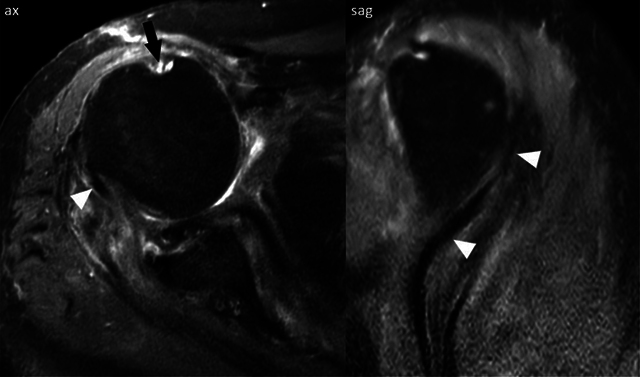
Axial and sagittal fat-saturated proton density-weighted MRI images showing a complete rotator cuff tear, an empty bicipital groove (arrow), and dislocation of the intact long head of biceps tendon (LHBT) posteriorly to the humeral head (arrowheads).
